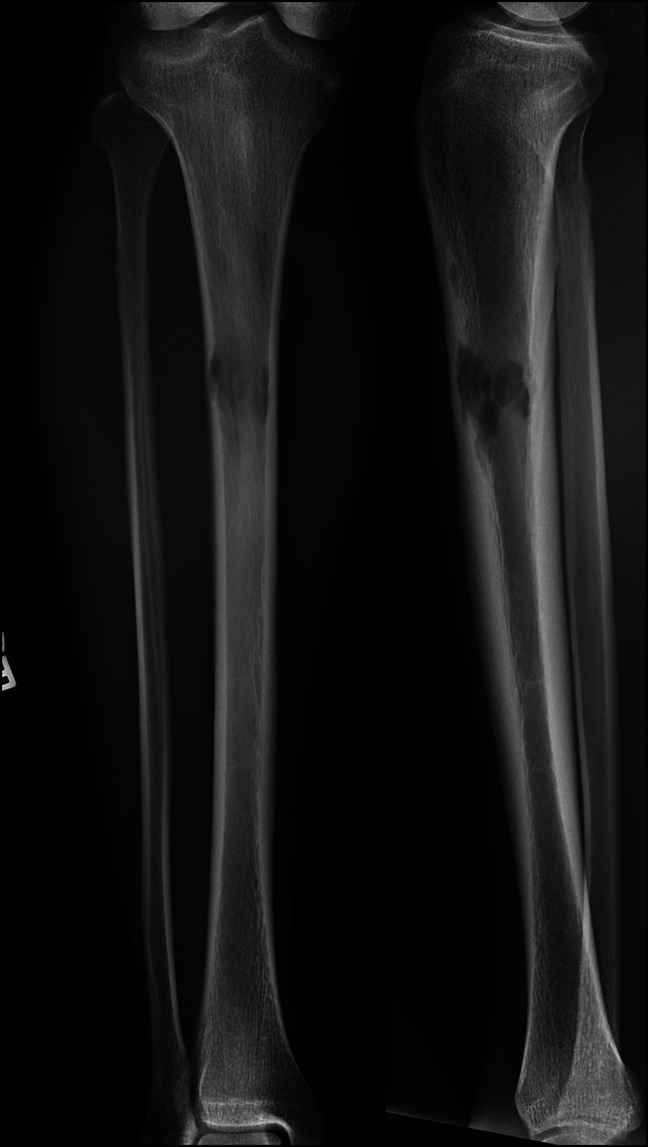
Radiograph of 27-year-old man demonstrating poorly defined lytic lesion in diaphysis of tibia with endosteal scalloping (case 1: AP and lateral radiographs of diaphyseal tibia lesion).
